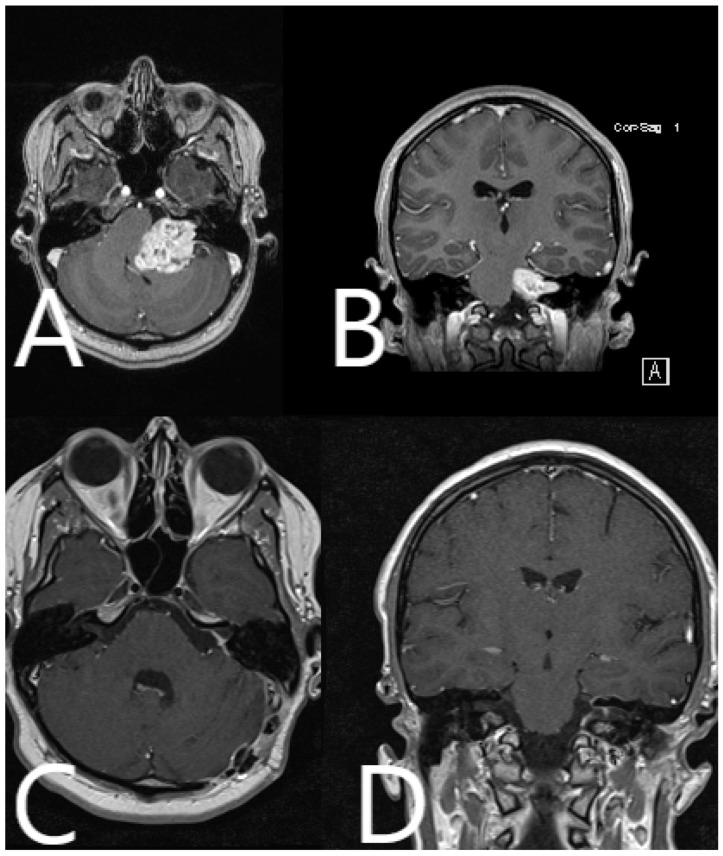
Patient No. 40. Preoperative (A) axial and (B) coronar T1-weighted post-contrast MRI with postoperative (C) axial and (D) coronar T1-weighted post-contrast MRI, depicting subtotal resection of the tumor and slight contrast enhancement along the course of the facial nerve.
